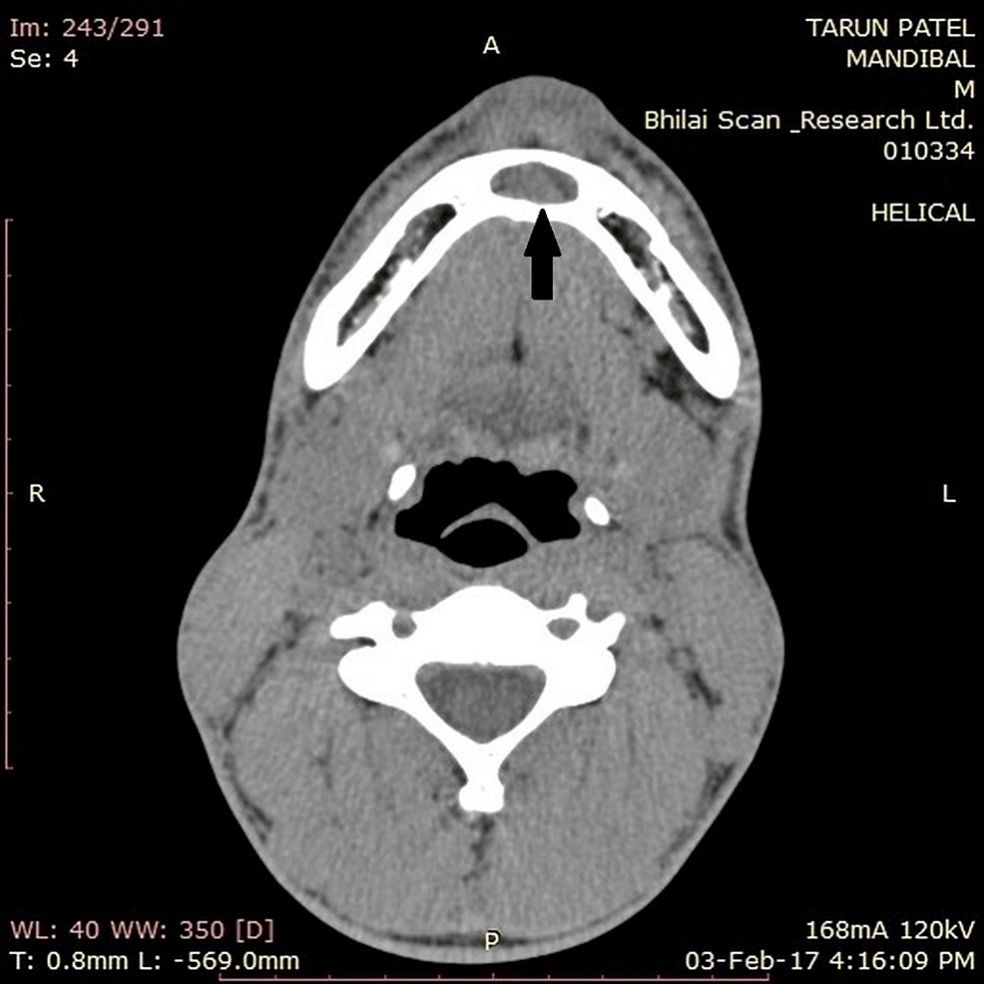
CT scan showing the cyst in the anterior region CT: computed tomography
